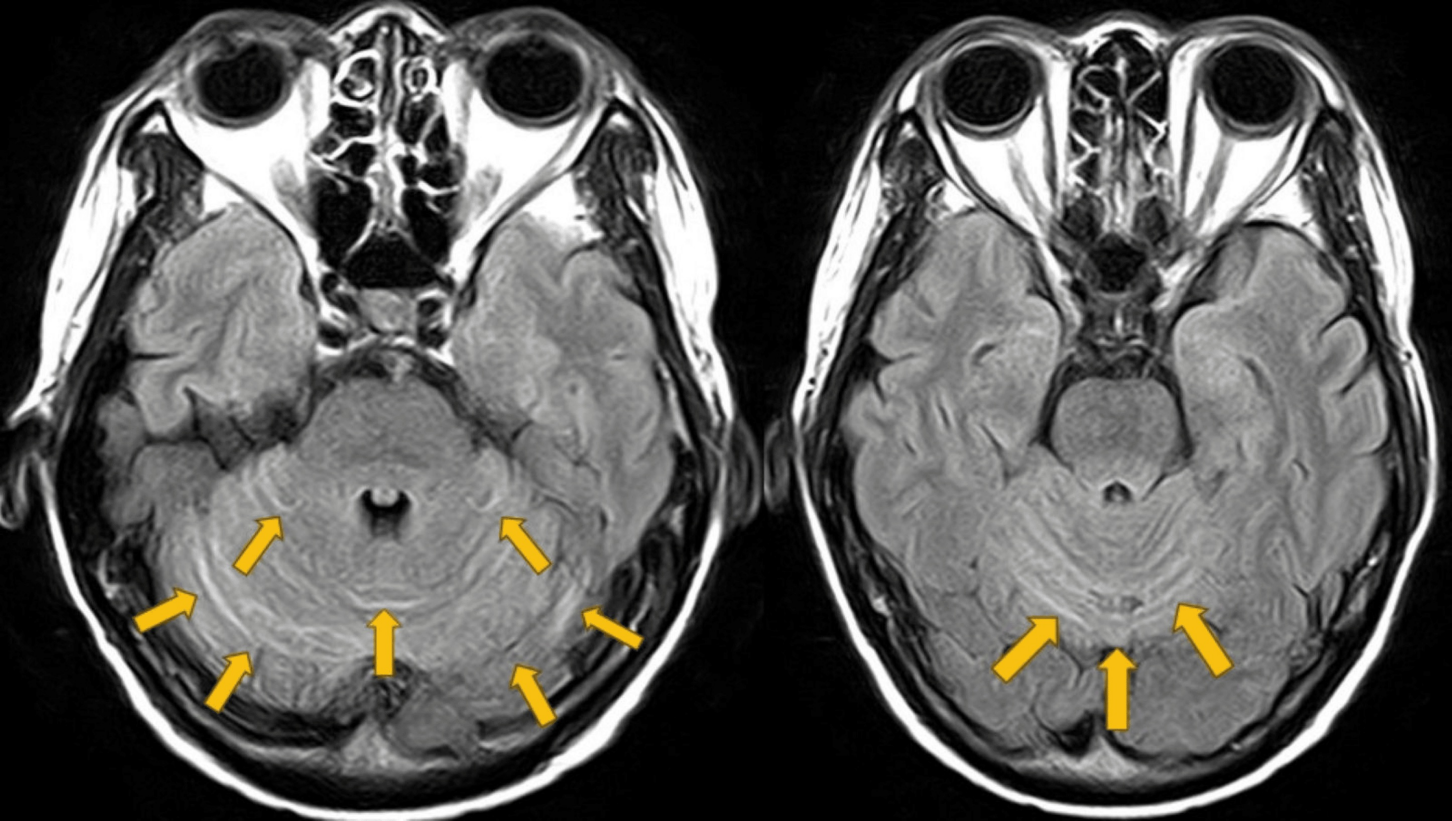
Contrast-enhanced T2-weighted MRI showing cerebellar high-signal intensity with prominent sulcal enhancement (yellow arrows) The observed contrast enhancement suggests the presence of inflammation in the affected area. MRI: magnetic resonance imaging
